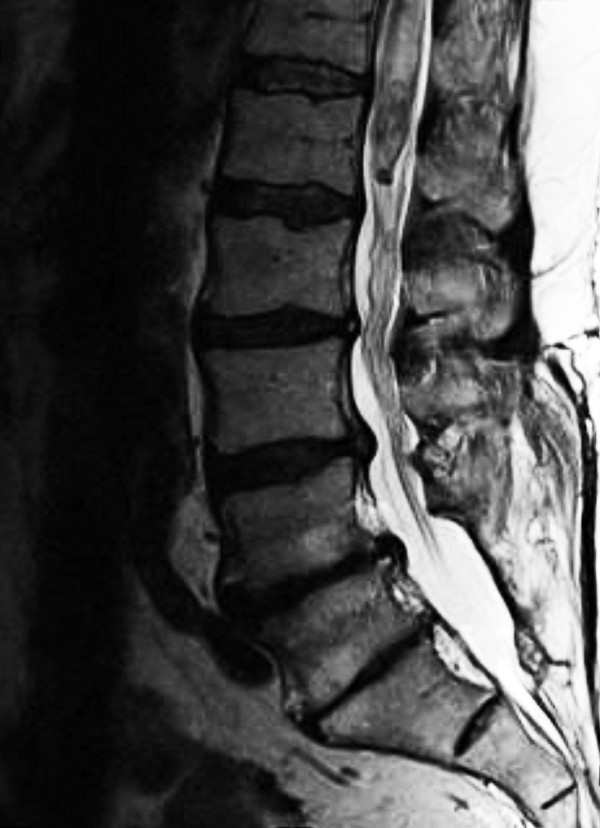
Sagittal T2-weighted magnetic resonance image.
